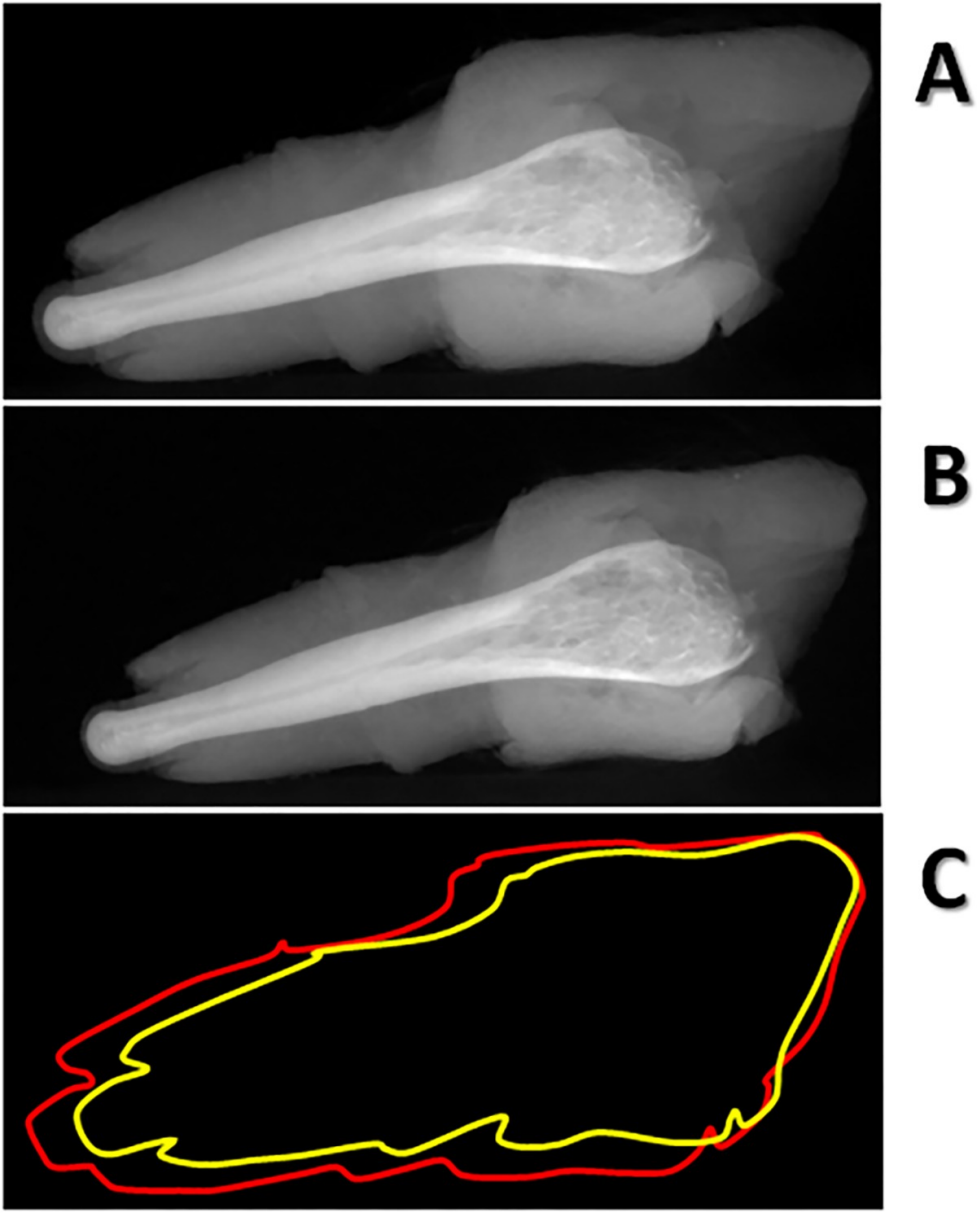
Sample volume reduction during the scan. Example of volume decrease occurred during the scan of a museum sample (*Loris lydekkerianus*) verified by comparing radiographic projection n° 0 (A) and n°899 (B) at the beginning and at the end of a tomographic acquisition process (900 radiographic projections acquired in an angular range of 360 degrees). The sample volume variation is shown (C) by overlaying outline of sample at the beginning of the scan (red line) and outline of sample at the end of the scan (yellow line).
